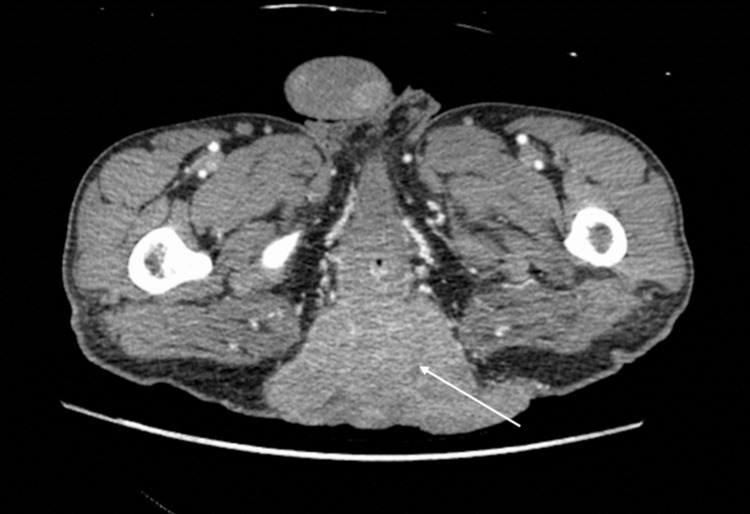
Abdominal and pelvic CT demonstrating a large fungating perianal mass abutting the anus.
